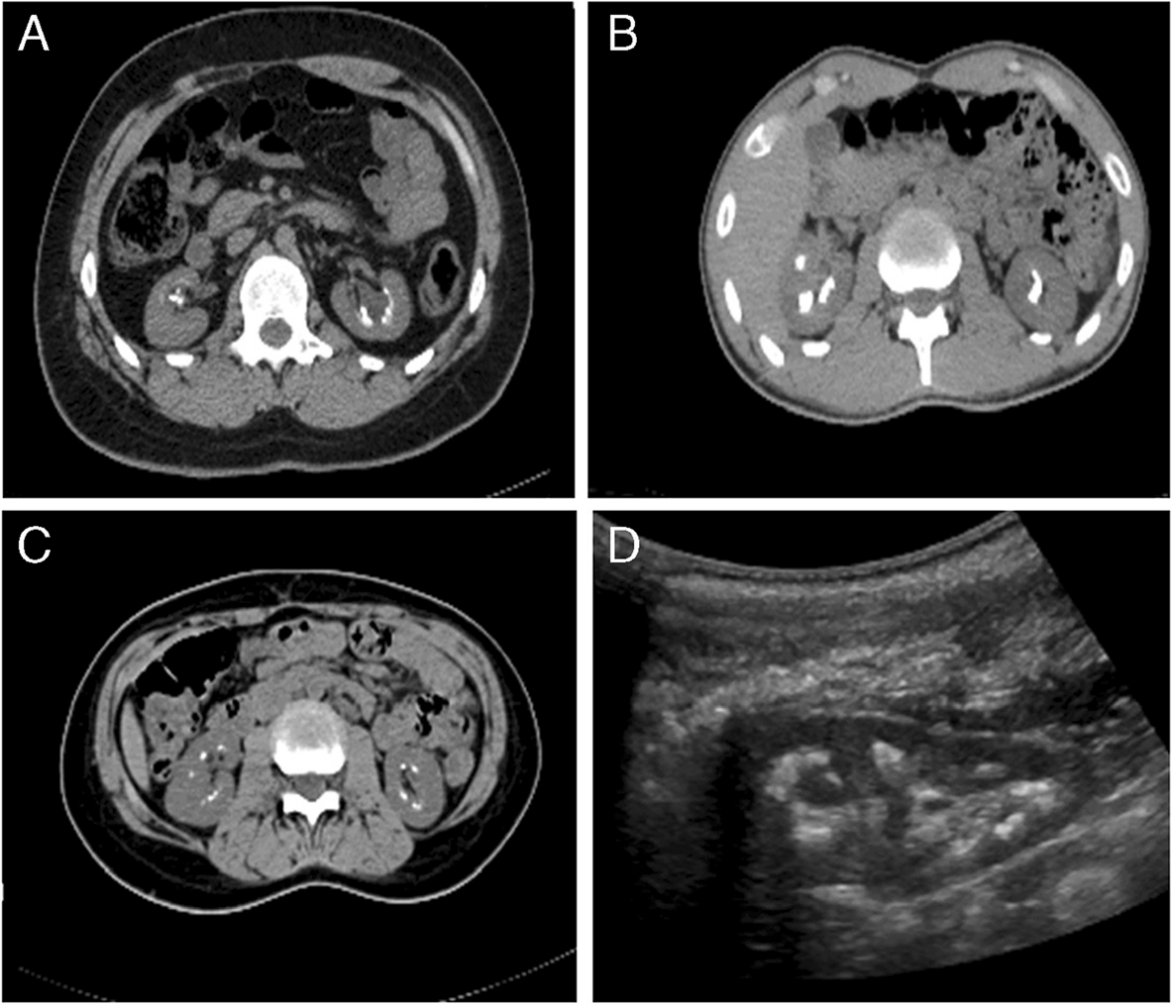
Imaging of the renal tract in affected members. A. CT scan showing bilateral nephrocalcinosis in II:1, aged 26 years. B. CT scan showing bilateral nephrocalcinosis in II:3, aged 22 years. C. CT scan showing bilateral nephrocalcinosis in II:8, aged 12 years. D. Renal USS demonstrating nephrocalcinosis in II:11, aged 3 years.
